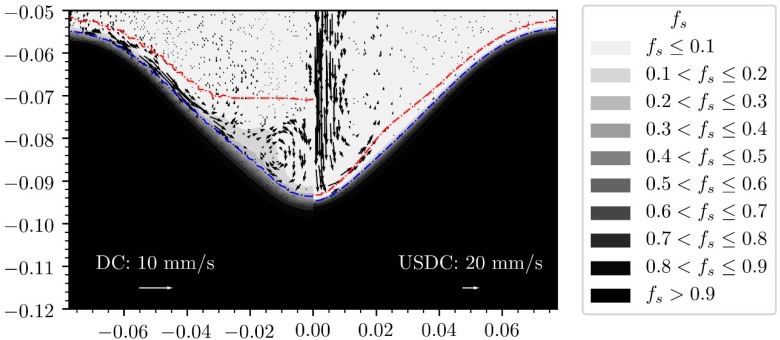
Comparison of sump profiles between conventional DC casting (left) and ultrasonic-assisted DC casting (right). $f_{s}$ is the solid fraction using the casting conditions defined in [38]. Arrows are shown for the scale of the velocity field. The red dash-dotted line represents the liquidus temperature and the blue dash-dotted line denotes the coherency temperature (solid packing fraction). The dataset used to reproduce these results is available elsewhere [73].
